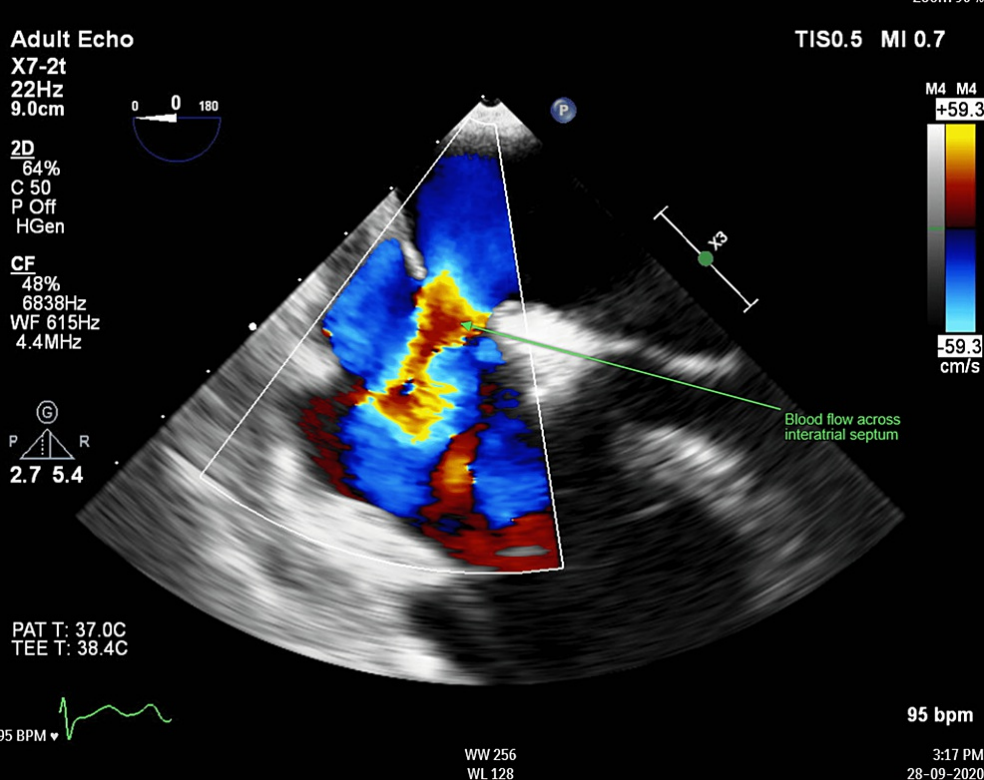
Transoesophageal echocardiogram demonstrating blood flow across the interatrial septum (green arrow)
